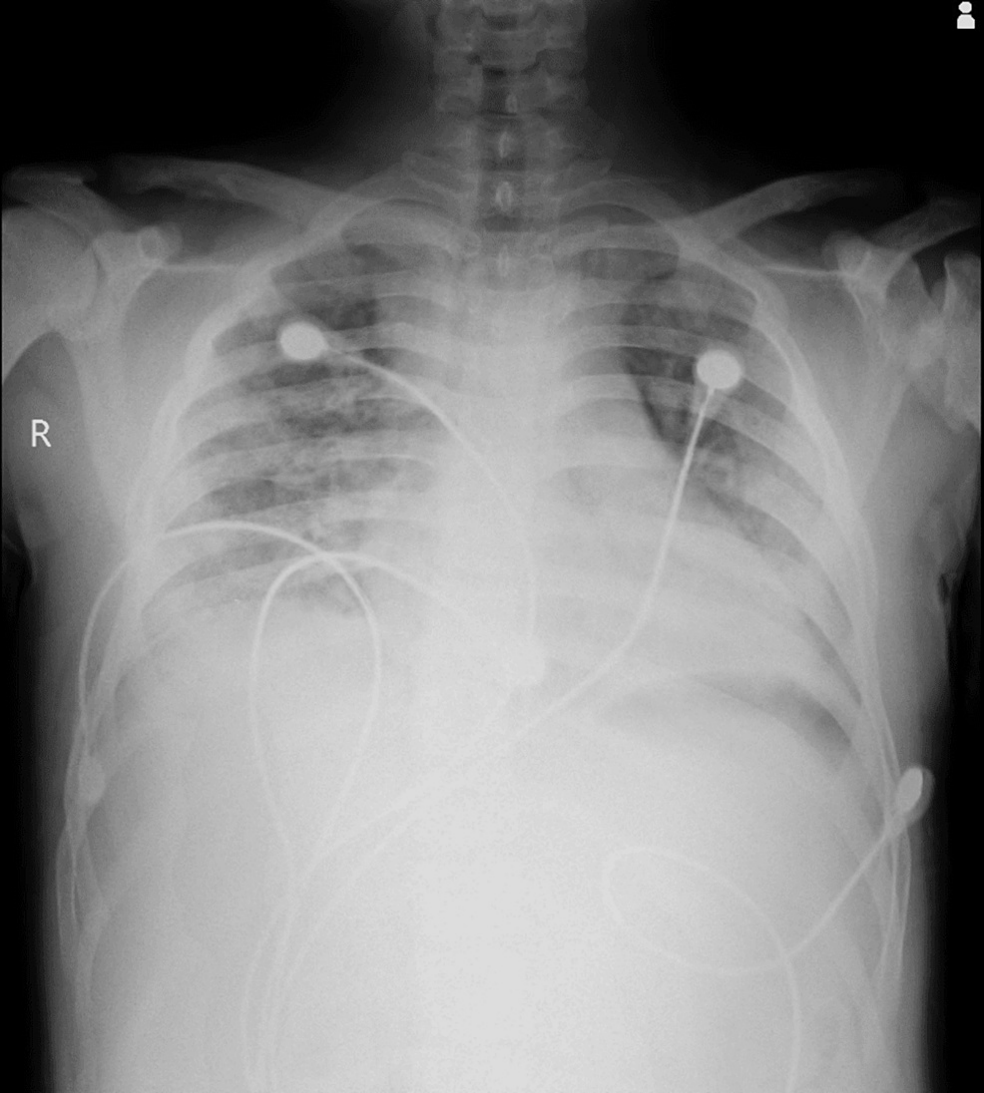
Chest X-ray of the patient on the third day since admission, suggestive of diffuse bilateral opacities throughout the lung fields
